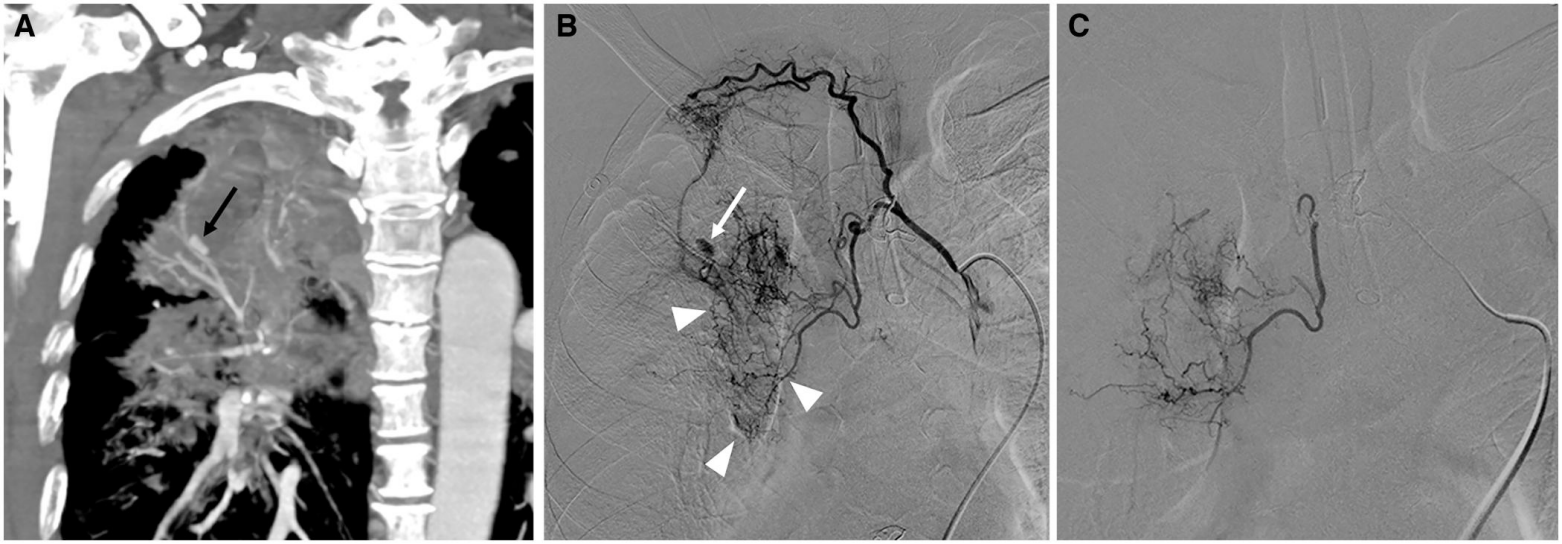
Rasmussen aneurysm in a 65-year-old man who presented with massive haemoptysis. (A) Coronal MIP CT image shows an aneurysm (black arrow) of a branch of the pulmonary artery in the right upper lobe. There is surrounding lung consolidation with areas of contrast extravasation. (B and C) Right intercostobronchial angiography was performed. (B) Preembolization image confirms the aneurysm (white arrow) and increased vascularity of the right upper lobe (white arrowheads). (C) Postembolization image shows occlusion of the aneurysm and reduced surrounding vascularity. (Courtesy of Dr. Tanop Srisuwan, Chiang Mai University, Thailand.)
